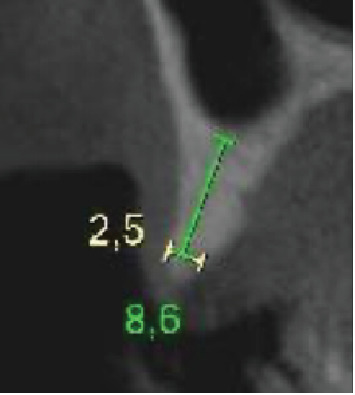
Initial CT of the region, highlighting measurements of 2.5 mm in thickness at the level of the crest and 8.6 mm in height.
